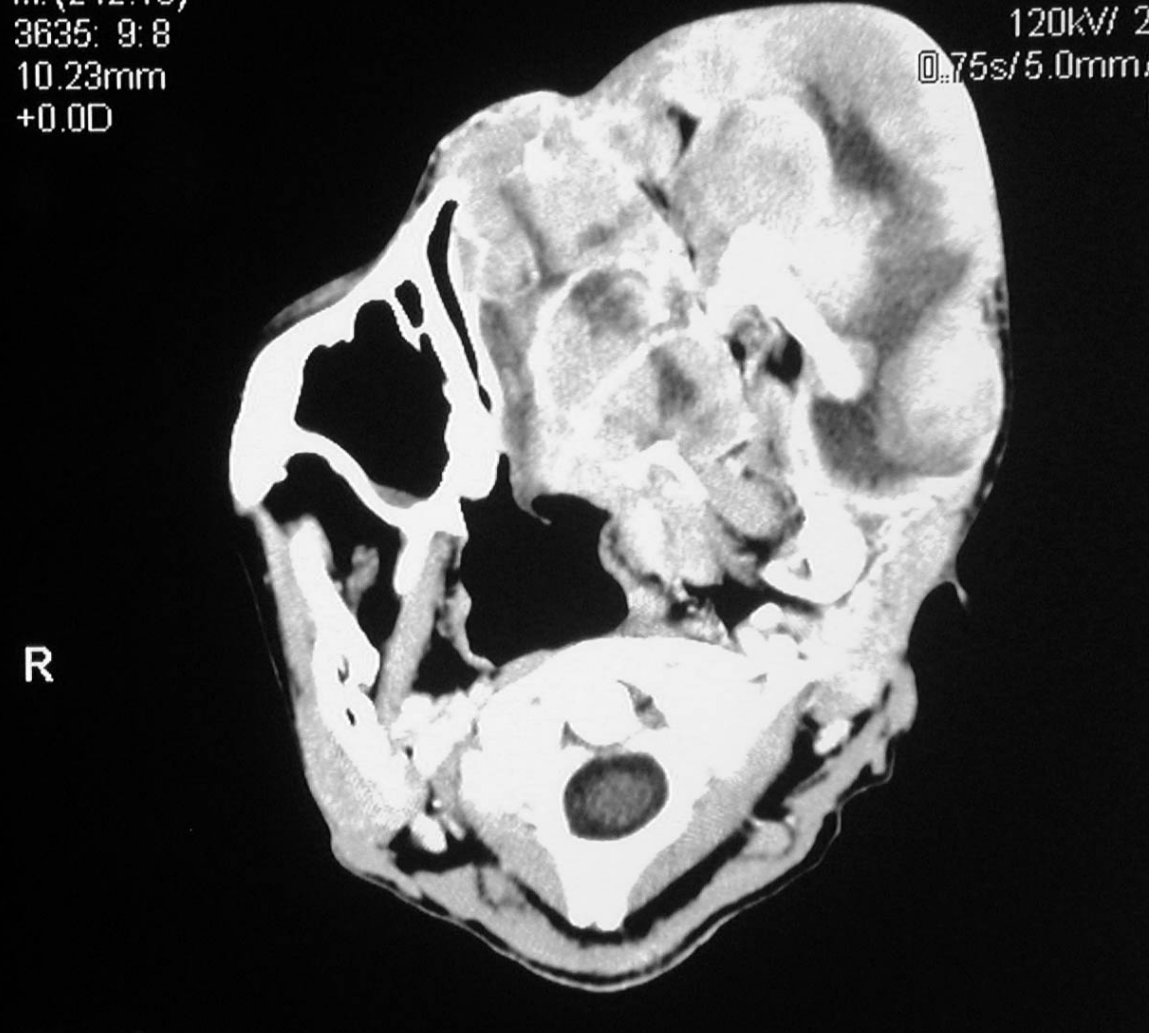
Axial CT cut showing the extent of the heterogeneous tumour.
